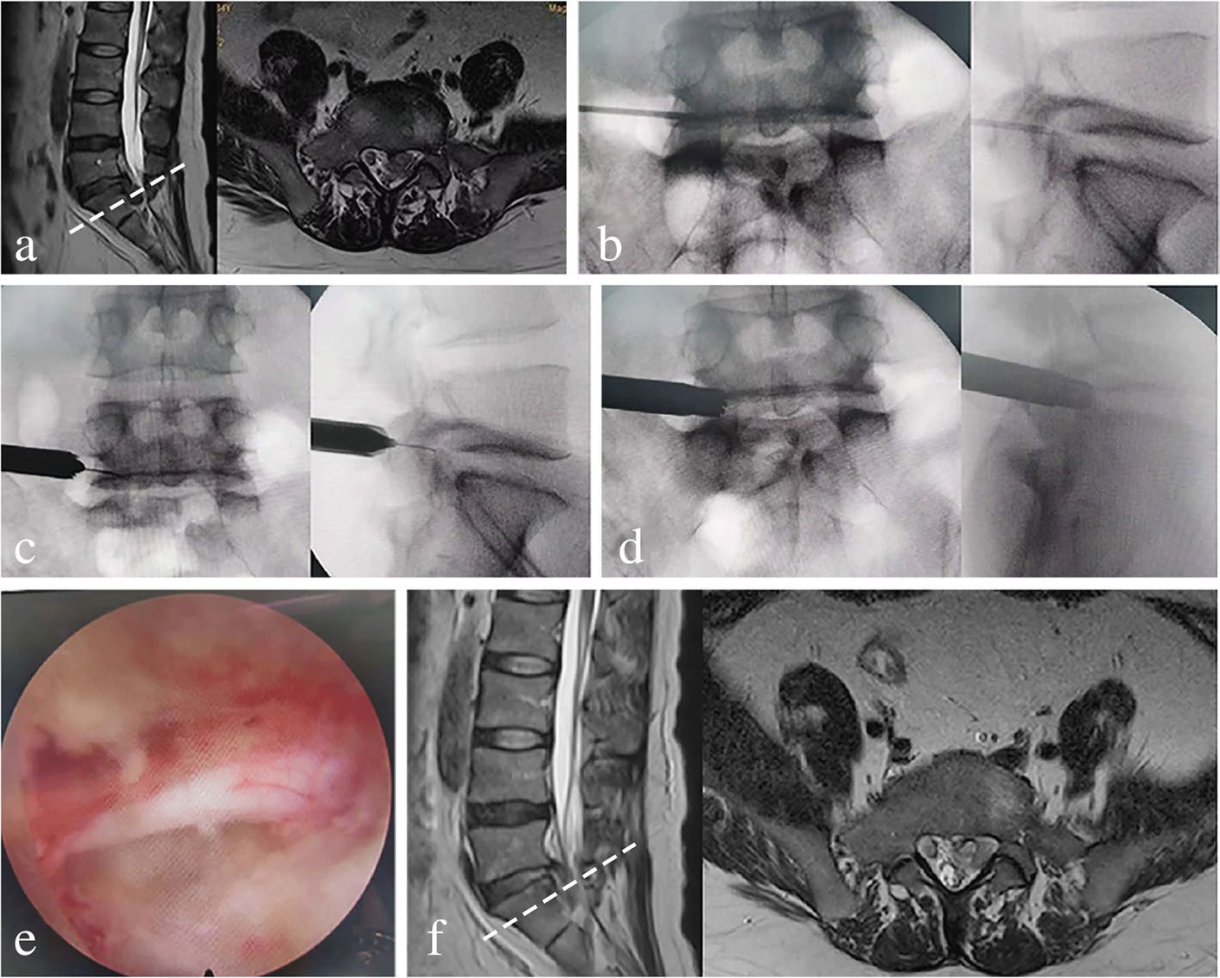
Thirty-four-year-old man with highly downward-migrated LDH, whose complaint was low back and left lower limb pain. The full-endoscopic foraminoplasty was performed. a Preoperative sagittal and axial T2 MRI showing a highly down-migrated disc from L5 to S1. b The needle tip located at the exterior margin and tip of the superior articular process. c The working cannula located at the exterior margin and tip of the superior articular process. d The ventral superior articular process was partially resected using the endoscopic trephine. e Thorough decompression was obtained. f Postoperative sagittal and axial T2 MRI showing successful surgical removal of the downward migrated fragments
